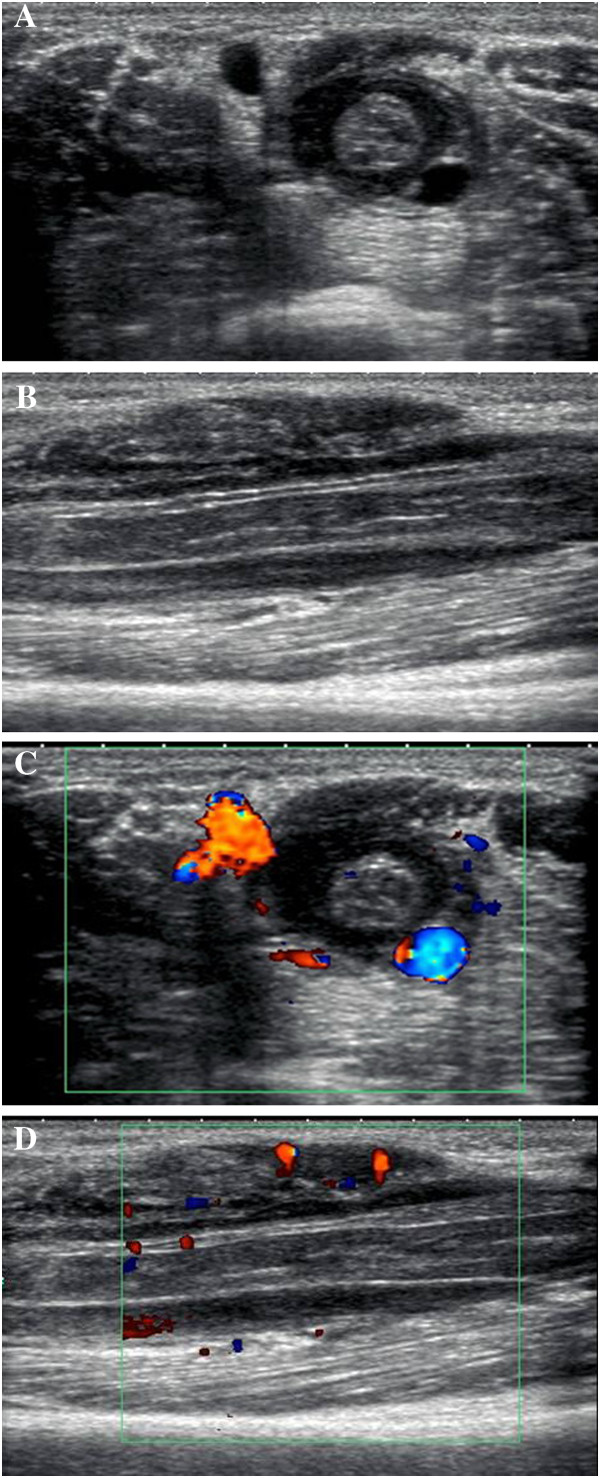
Sonography images demonstrated a lesion encased the swollen median nerve. (A) The transverse sonogram shows a round hypoechoic halo with well-defined margins and posterior acoustic enhancement completely surrounded the swollen median nerve. The brachial artery was partially encased, while the basilic vein and ulnar nerve were unaffected. (B) The longitudinal sonogram shows the mass extended along the median nerve. The swollen nerve shows hypoechoic echotexture lesion with discernible fascicles. The adjacent biceps brachii muscle with inhomogeneous-reduced echogenity is noted. (C) Avascularity could be visualized on the color Doppler image inside the mass. (D) Slightly increased vascularity is observed on color doppler sonography in biceps brachii muscle adjacent to the lesion.
